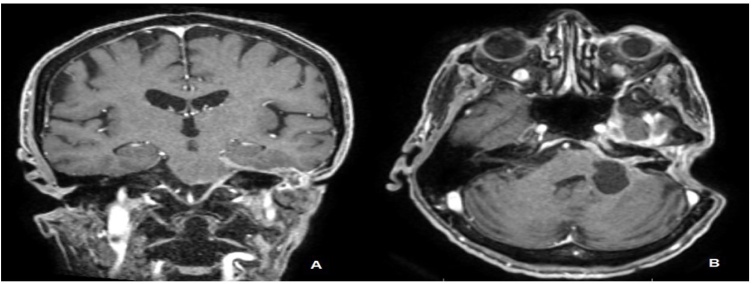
Magnetic resonance imaging of a T1-weighted skull. Paquimeningeous thickening along the cistern of the left cerebellar point angle, as well as the interior of the internal auditory canal on the left side, is pictured. It is associated with an expansive lesion, apparently cystic, in continuity with the tissue observed inside the left internal auditory canal, measuring approximately 3.1 × 1.9 cm in the largest axes in the axial plane. (A) Coronal cut. (B) Axial cut.
